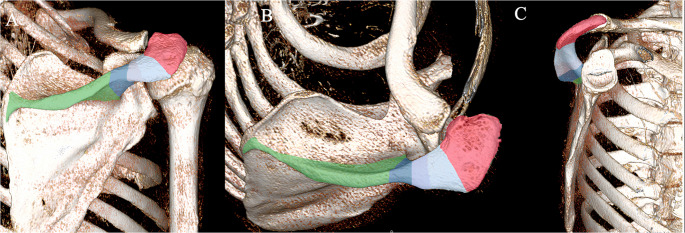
Modified Levy Classification in coronal (A), axial (B), and sagittal (C) 3-dimensional CT reconstructions. Zone I fractures (red) occur anterior to the posterolateral corner of the acromion. Zone II fractures (blue) occur between the posterolateral corner of the acromion and its base and are further divided into IIA (pale blue), IIB (periwinkle), and IIC (navy) subtypes. Zone III fractures (green) involve the scapular spine
